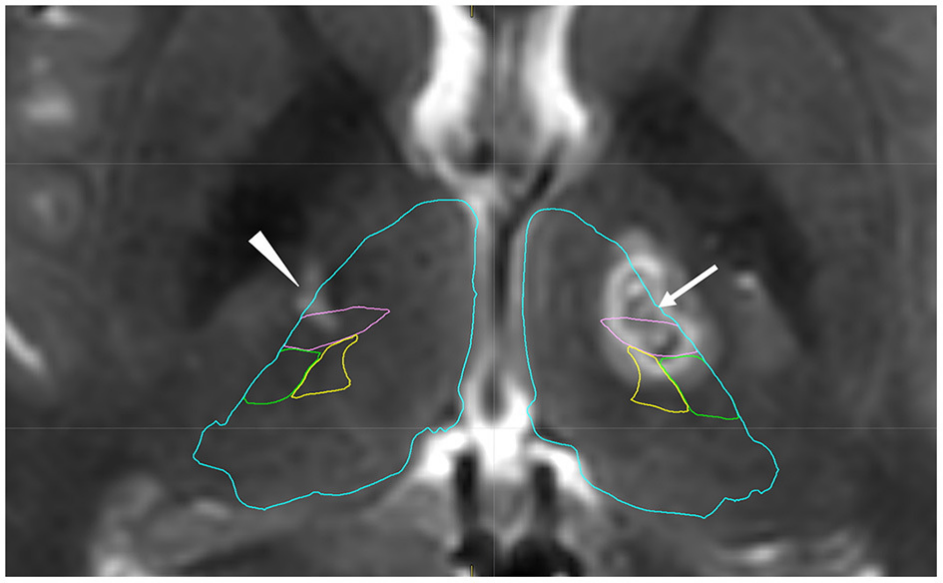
Postoperative T2-weighted image after left thalamotomy with anatomical mapping by Brainlab Elements. The arrow shows coagulated lesions in the left ventro-oral (Vo) nucleus. Posterior coagulated lesions are located in the ventral intermediate nucleus (Vim). The arrowhead shows an old lesion after previous surgery, which was confirmed in the Vo and Vim nucleus. Blue: thalamus, Pink: Vim, Green: ventral posterior lateral nucleus, Yellow: ventral posterior medial nucleus.
